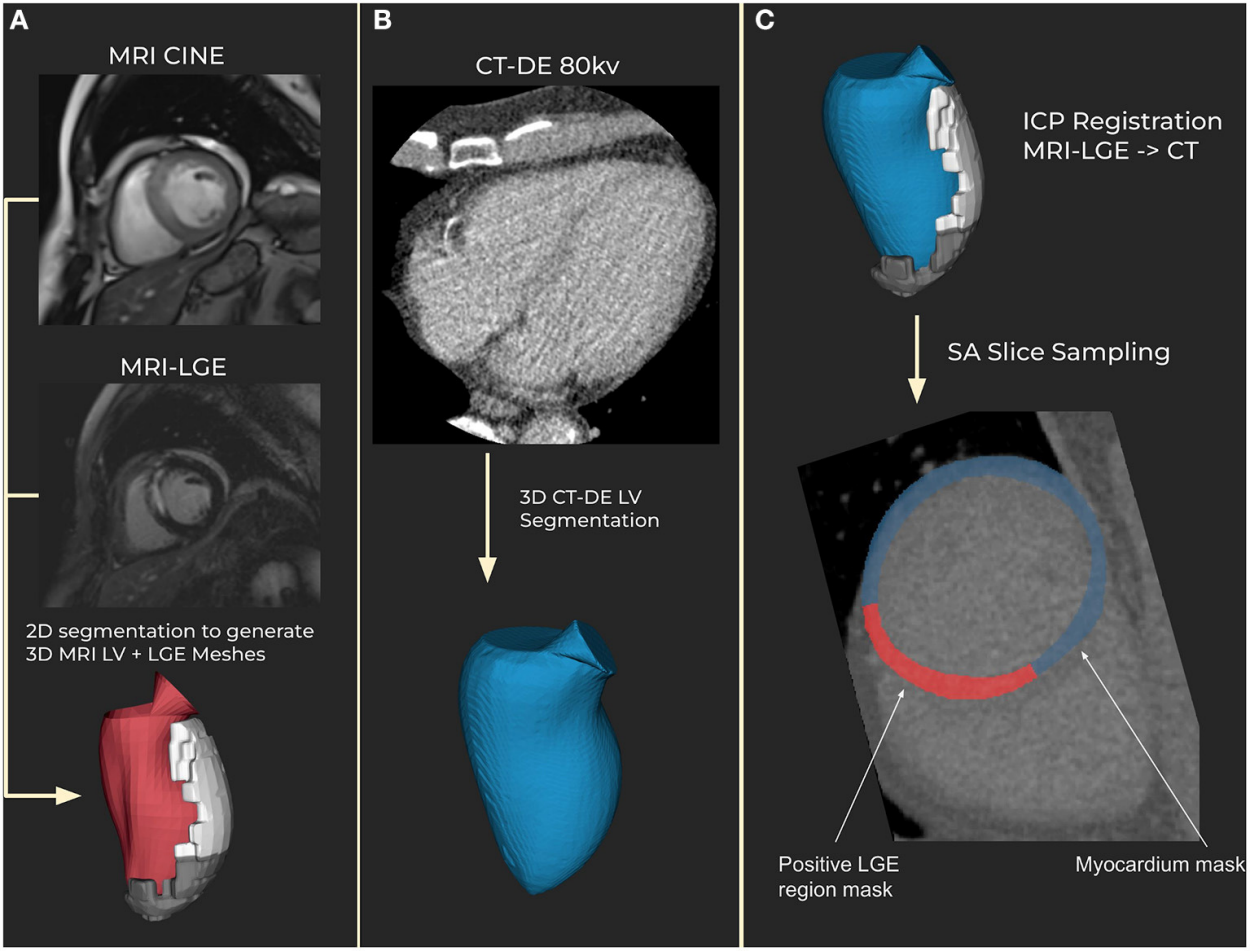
Segmentation and registration. (A) Magnetic resonance imaging (MRI) segmentation using CINE MRI for anatomical 3D mesh and MRI late gadolinium enhancement (LGE) for scar mesh. Endocardial (red) and scar meshes (white) shown (B) Delayed enhancement computed tomography (CT-DE) segmentation to generate a 3D mesh of left ventricle from CT. CT endocardial mesh is shown in blue. (C) CT scar mesh generated by iterative closest point (ICP) registration from the MRI to CT left ventricle anatomical meshes. Applying the resulting transform to the MRI-LGE mesh produces a CT aligned scar mesh. This provides locations to mask for scar on the CT-DE.
